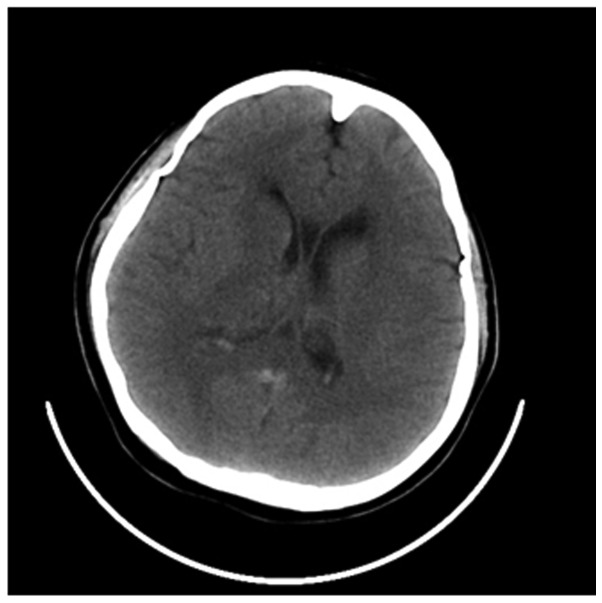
Computed tomography (CT) showed a high density vein of Galen and right thalamus swelling with decreased density.
